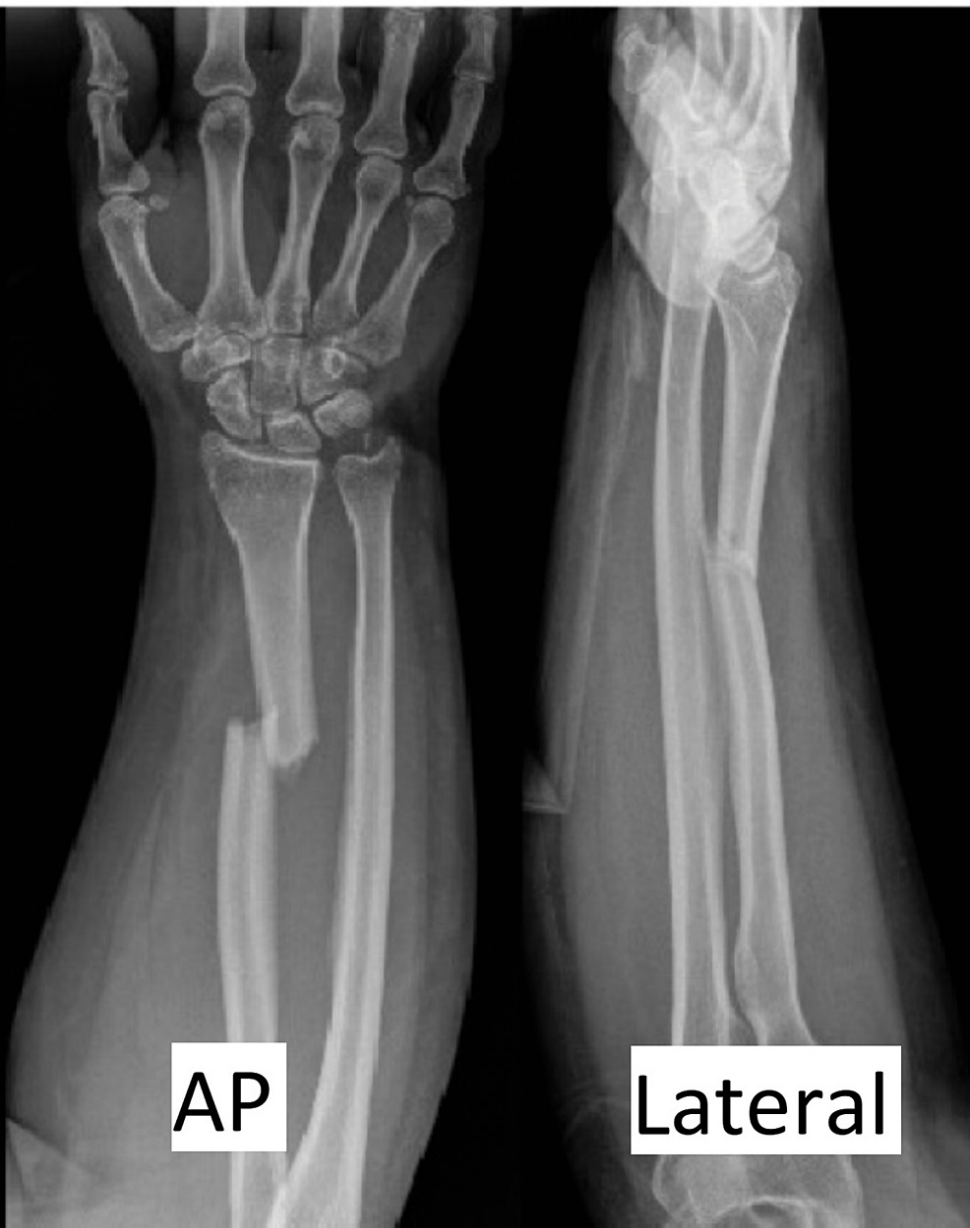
Preoperative anteroposterior (AP) and lateral view X-rays of the right forearm demonstrating distal-third radial shaft fracture and associated ulnar styloid fracture
